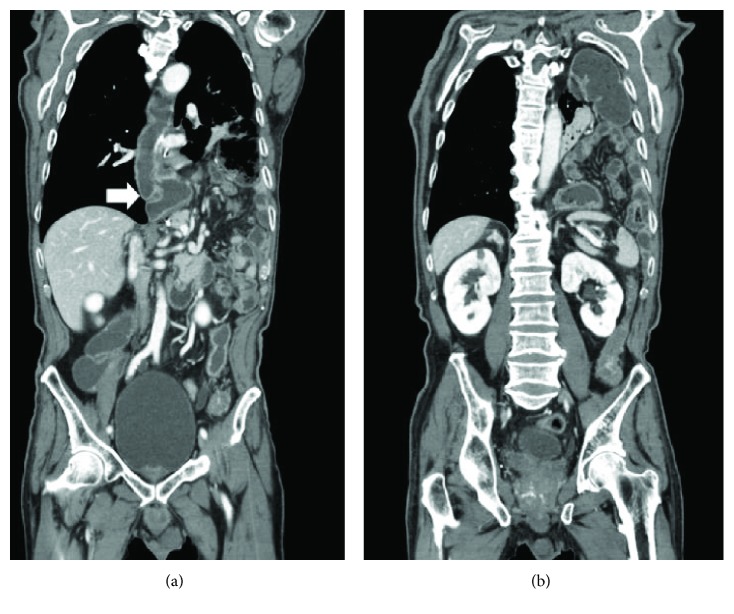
Coronal CT scan showing the massive transdiaphragmatic herniation of abdominal viscera in the left hemithorax. (a) The gastric conduit on the right of the herniated viscera (arrow). (b) The left hemithorax totally occupied by the abdominal viscera.
